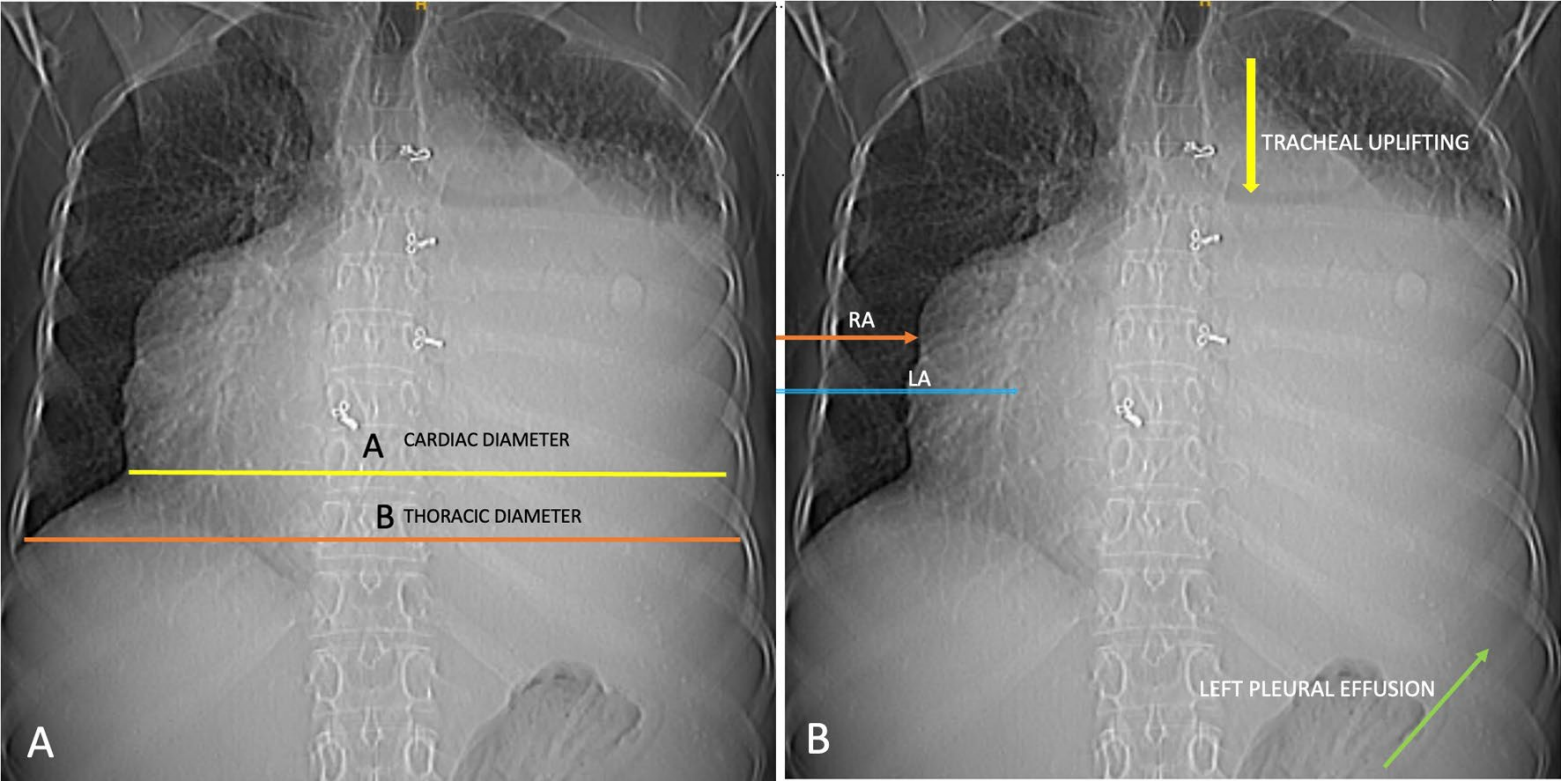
Chest X-ray. A Chest X-ray shows cardiothoracic ratio of 0.88 and B chest X-ray demonstrating dilated right atrium (RA), dilated left atrium (LA), tracheal uplifting due to LA enlargement, and left pleural effusion
